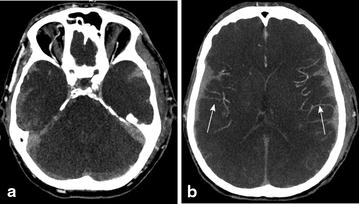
CTA axial views of a clinically brain-dead patient with a lack of SPV opacification (a) despite an enhanced bilateral C-MCA (b) (*arrows*)
